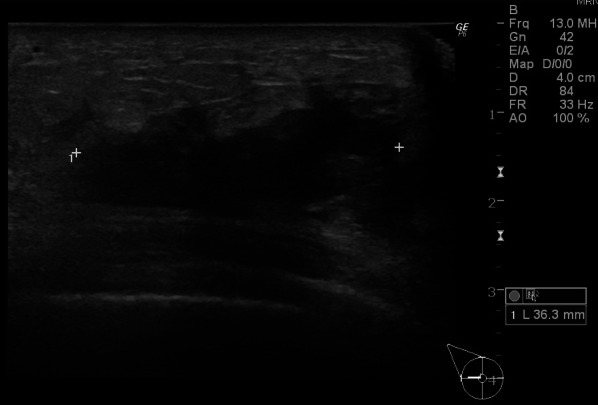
Breast ultrasonography revealed a 36-mm irregular mass at the 9 o’clock position
